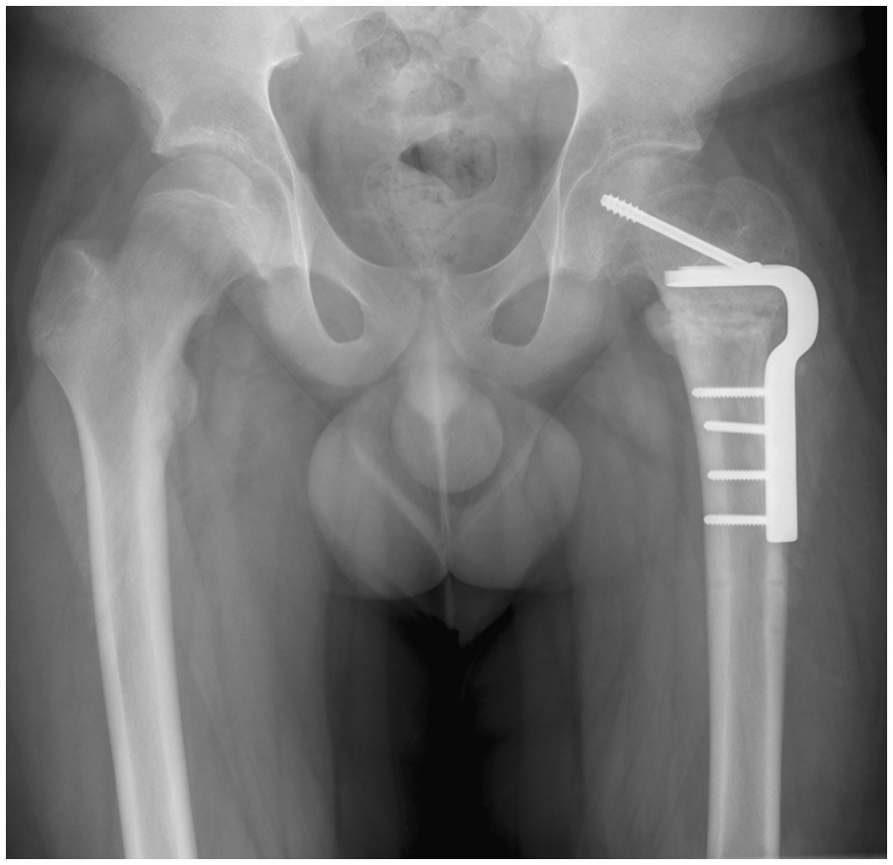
Patient 1. The anteroposterior X-ray after the Southwick correction osteotomy with screw fixation of the head.
